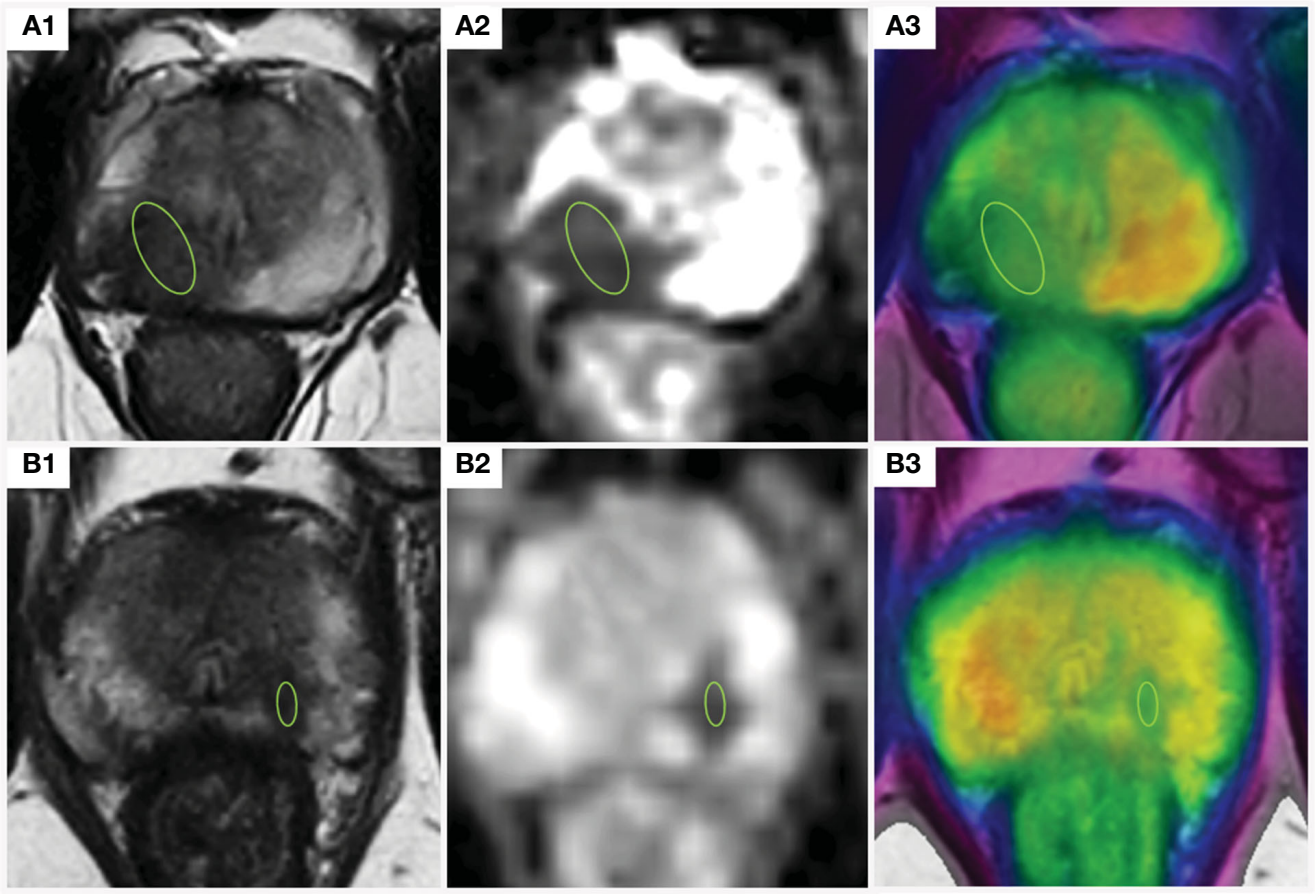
Indications of the definition of the ROIs for parameter analyses. (A) prostate cancer with EPE; A1,2: The lesion appeared hypointense on the T2-weighted image and the ADC map, ADC =0.92×10^−3^ mm^2^/s; A3: APT-weighted image with a T2WI overlay (APT =3.76%); (B) prostate cancer confined within the organ: B1,2: The lesion appeared hypointense on the T2-weighted image and the ADC map, ADC =1.26×10^−3^mm^2^/s; A3: APT-weighted image with a T2WI overlay (APT =3.32%); ADC, apparent diffusion coefficient; APT, amide proton transfer; TEPE, Extraprostatic extension; ROI, region of interest. T2WI, T2-weighted imaging.
